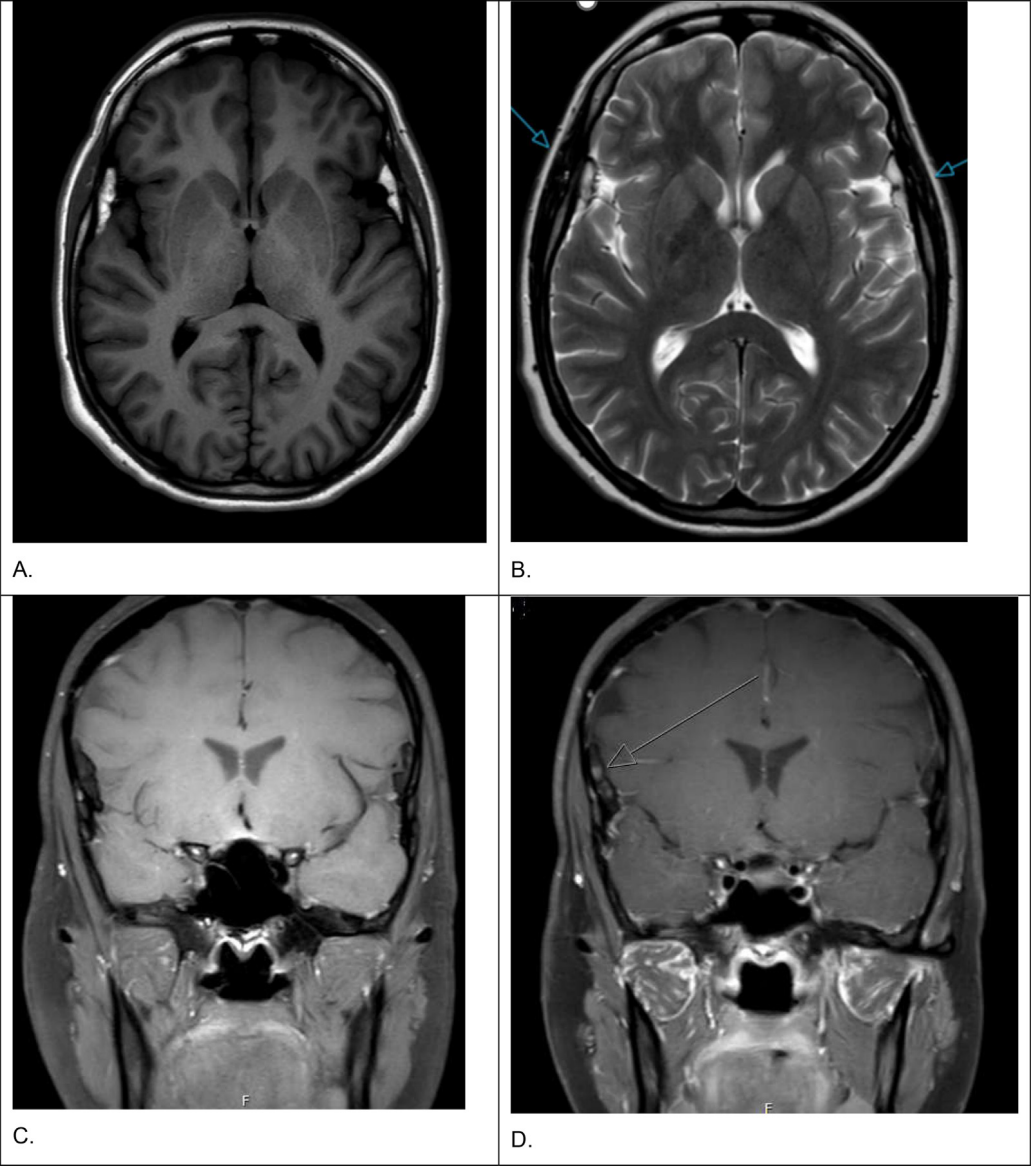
MRI of the bilateral sylvian fissure lipomas with angiomatous component. MR images at the level of the Sylvian fissure with axial T1-weighted sequence (A), axial T2-weighted sequence (B), coronal pre- and postcontrast T1-weighted sequence with fat suppression technique (C and D). The images show bilateral perisylvian lesions with hyperintense signal on the T1-weighted sequence (A), with hypointense signal with fat suppression technique on the T1-weighted sequence (C and D) indicating that the lesions contain fat. On the T2-weighted sequence the lesions are also hyperintense (B). After administration of gadolinium, the lesions show partially enhanced signal (arrow) on T1-weighted imaging, indicating an angiomatous component of the lesions (D).
